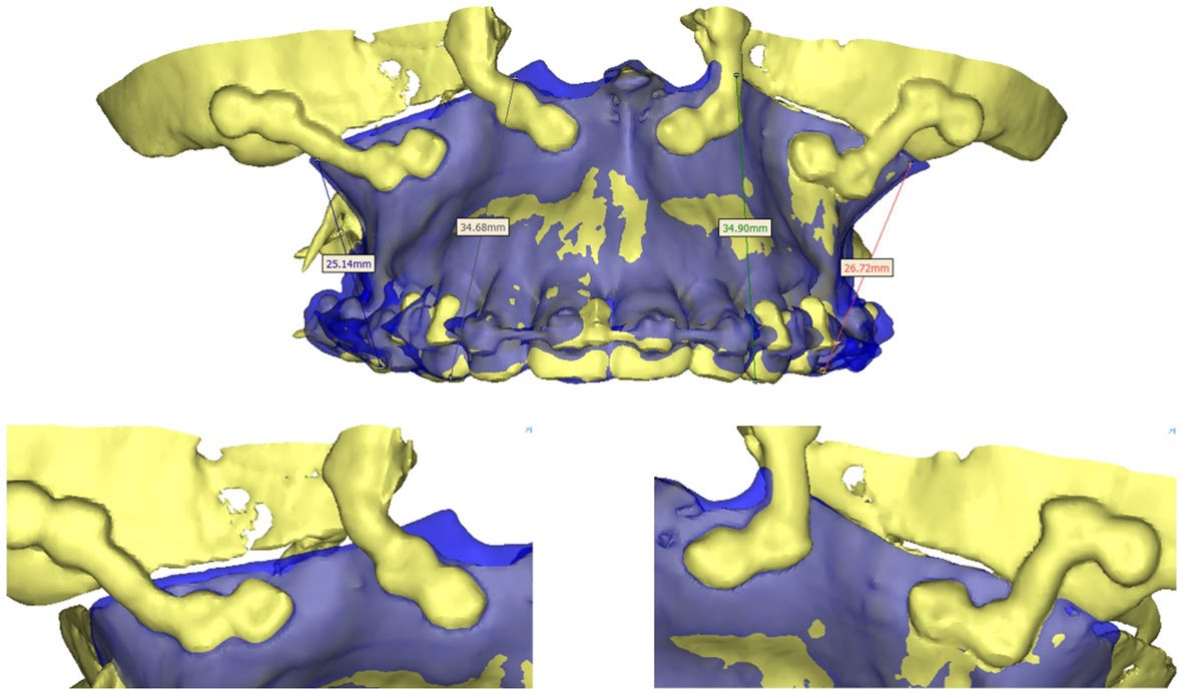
Overlay of preoperative planned, cut maxillary segment (blue overlay) with postoperative outcome of the osteotomy (yellow) for a patient with pre- and postoperative CT images.
